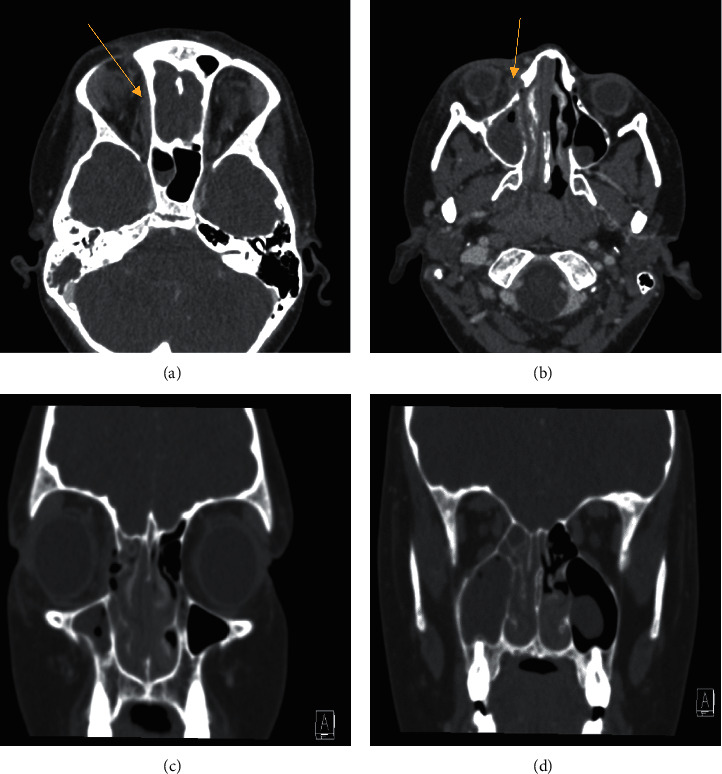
Computed tomography with contrast images of the presented case: (a) axial cut demonstrating a superior and medial subperiosteal abscess (yellow arrow). (b) a smaller rim-enhancing fluid collection inferior to the abscess in A. (c) & (d) coronal sections through the paranasal sinuses with notable mucosal thickening and mucosal thickening with air-fluid levels within the right maxillary sinus, right ethmoid air cells, and right sphenoid sinus.
